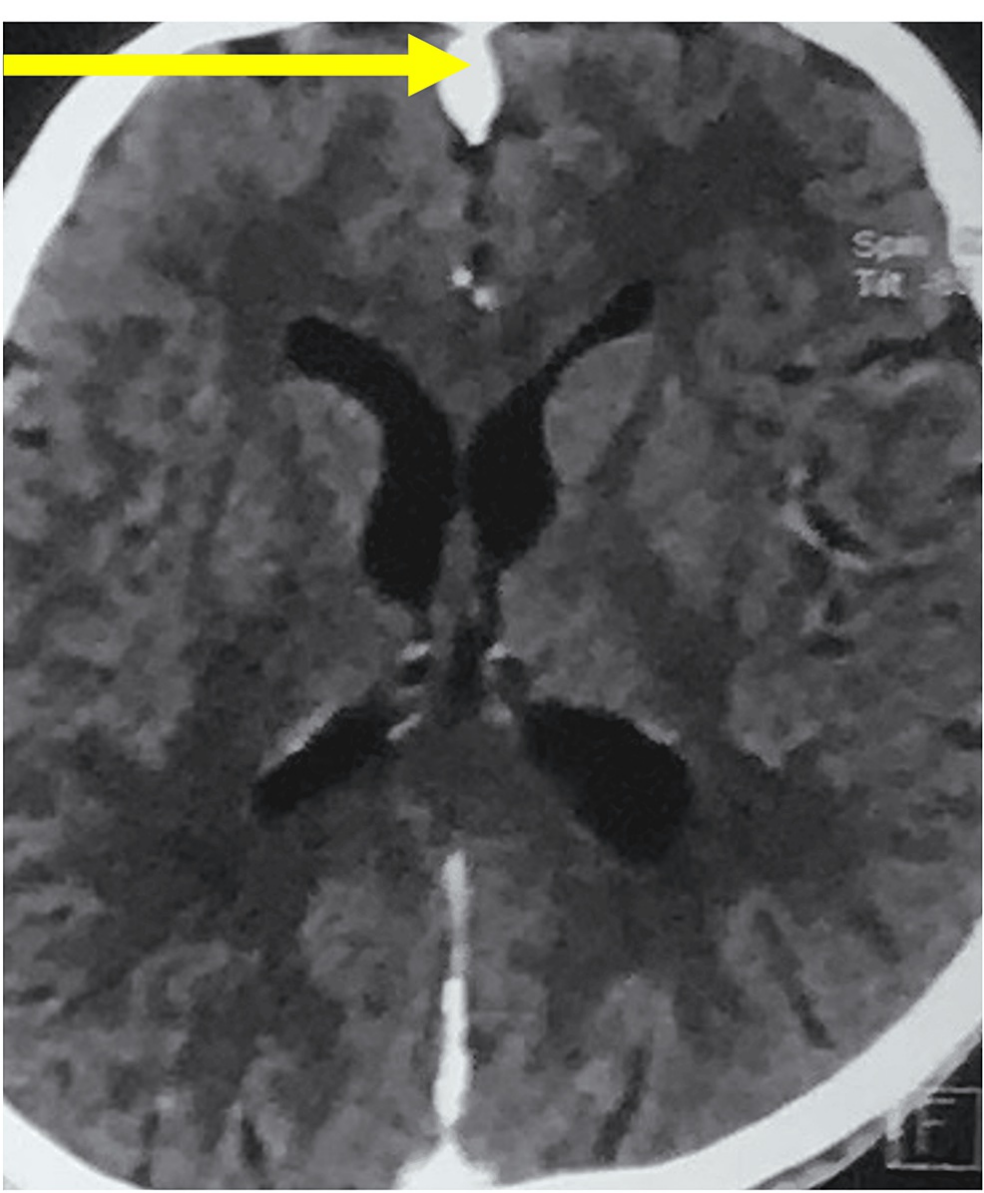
CT Axial section brain showing calcification of falx cerebri
